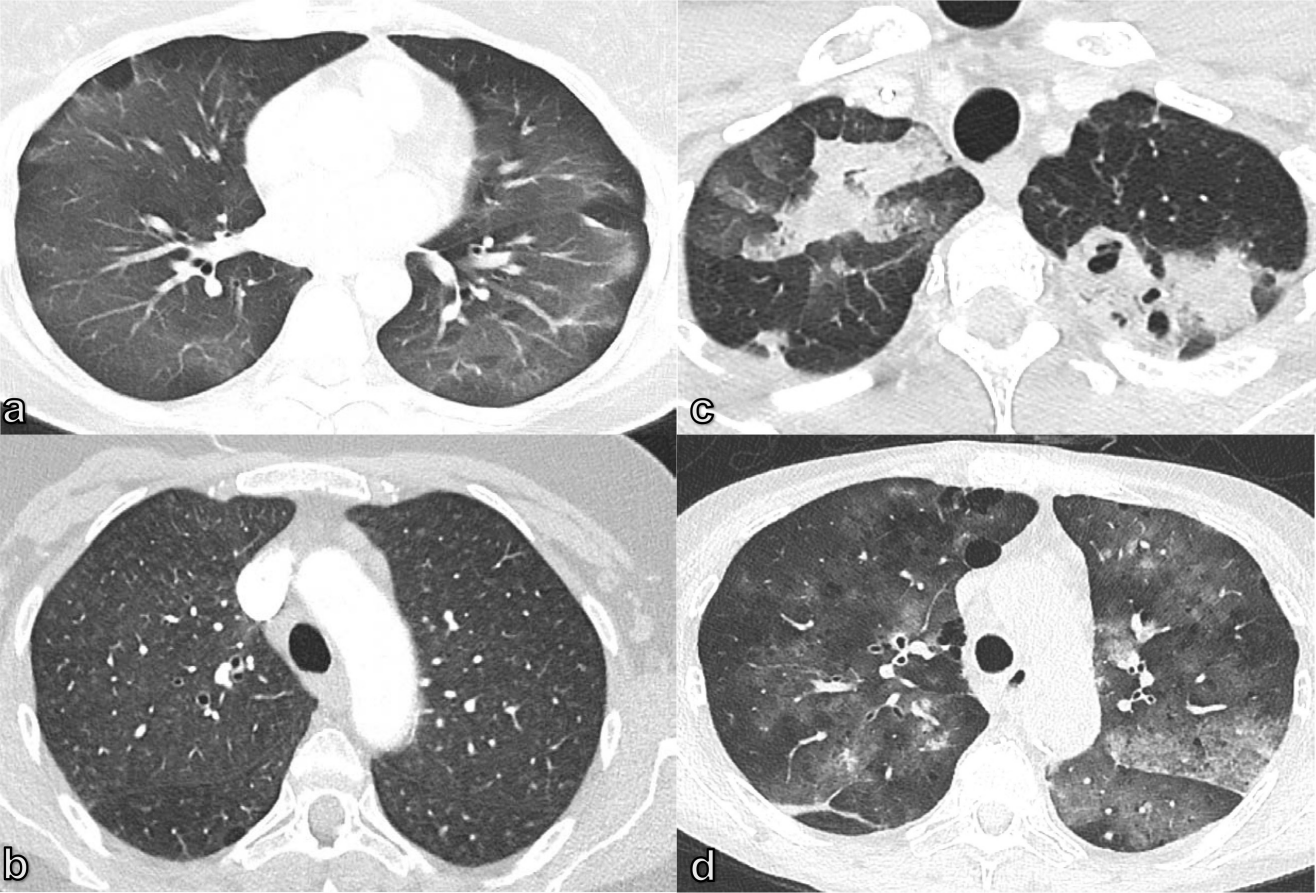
Diagnostic chest CT in dyspneic patients: (a) 48-year-old female patient with inhalation trauma and extensive ground-glass opacities as signs of permeability (non-cardiogenic) edema; (b) 52-year-old female patient with ill-defined ground-glass centrilobular nodules indicative of hypersensitivity pneumonitis; (c) 36-year-old male patient with endocarditis after tricuspid valve repair, *Staphylococcus aureus* sepsis and septic emboli; (d) 61-year-old female patient with lung hemorrhage as a result of systemic sclerosis vasculitis.
